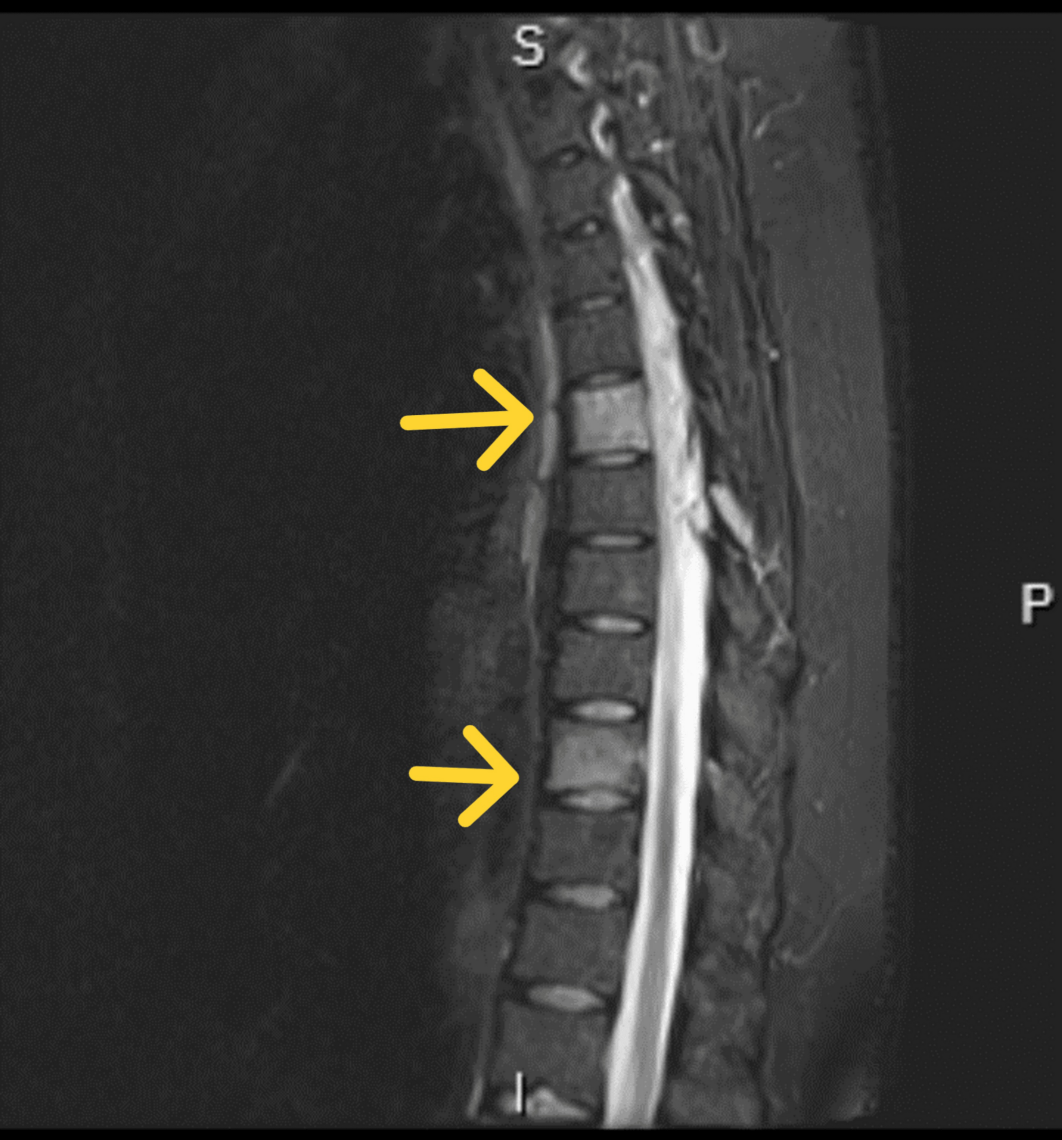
Sagittal MRI of the thoracic spine (T2-weighted).
